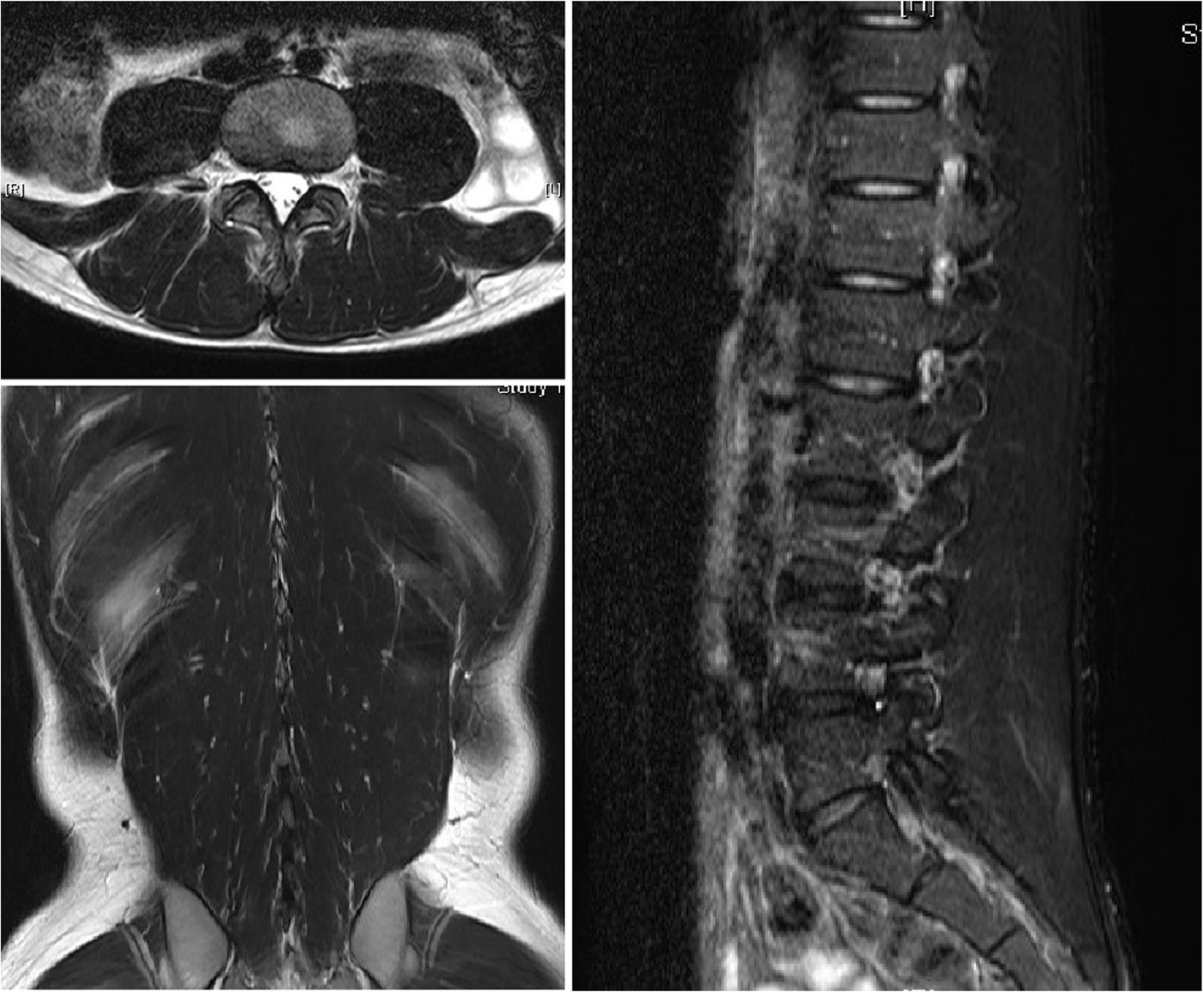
Magnetic resonance imaging after 3 months. No change was noted
